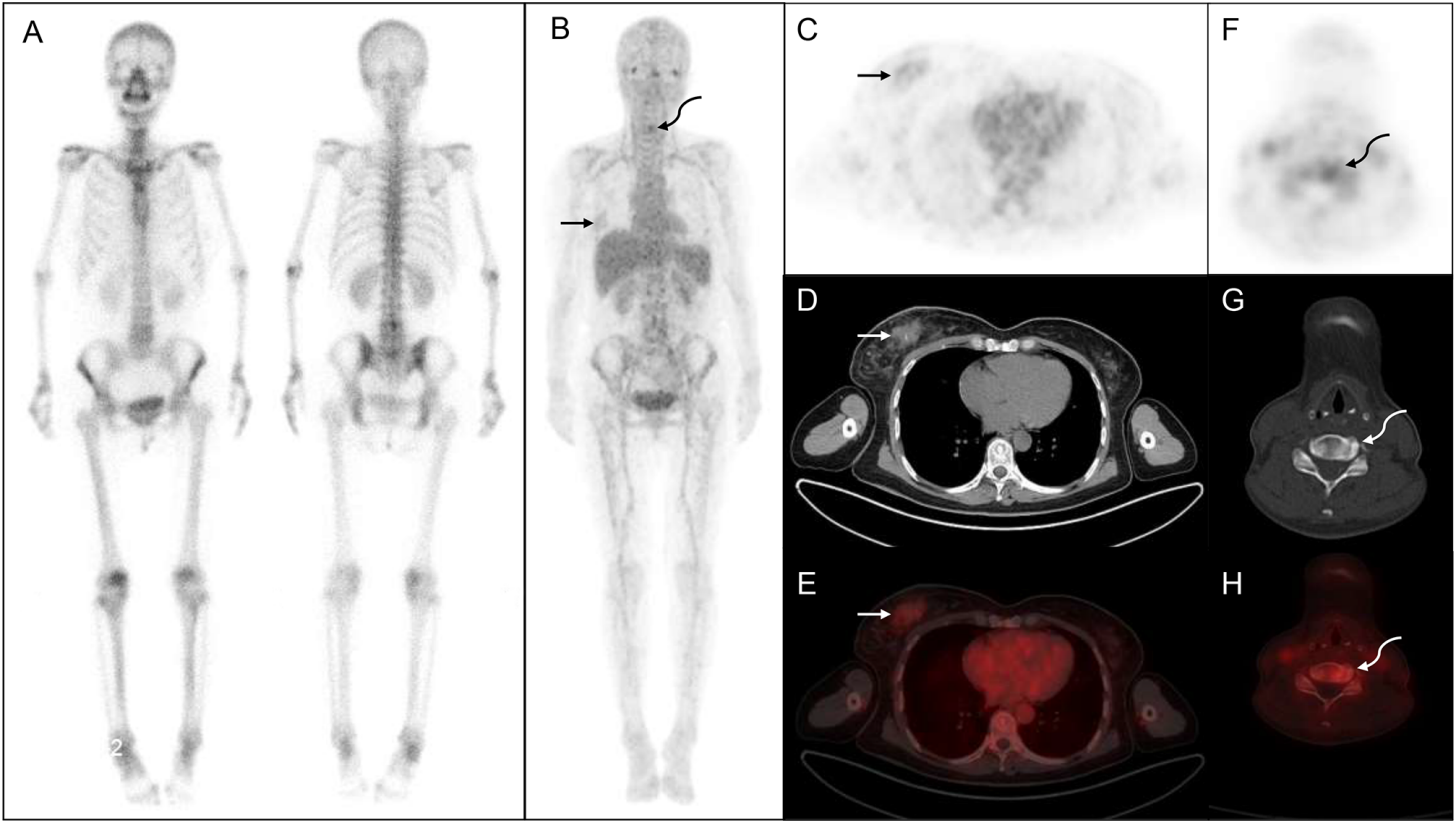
^68^Ga-DOTA- ibandronate (IBA) PET/CT performed on a 54-year-old woman with breast cancer. A ^99m^Tc-MDP bone scan (A) shows no significant abnormalities, whereas a ^68^Ga-DOTA-IBA PET/CT (B) shows increased tracer uptake in the right mammary gland (straight arrow) and cervical spine (curved arrow). (C–E) shows right breast nodules with abnormal tracer uptake (SUVmax, 1.84; straight arrow). (F–H) shows a cervical bone change with increased metabolism (SUVmax, 3.46, curved arrow) that is subsequently confirmed as invasive cancer via biopsy.
